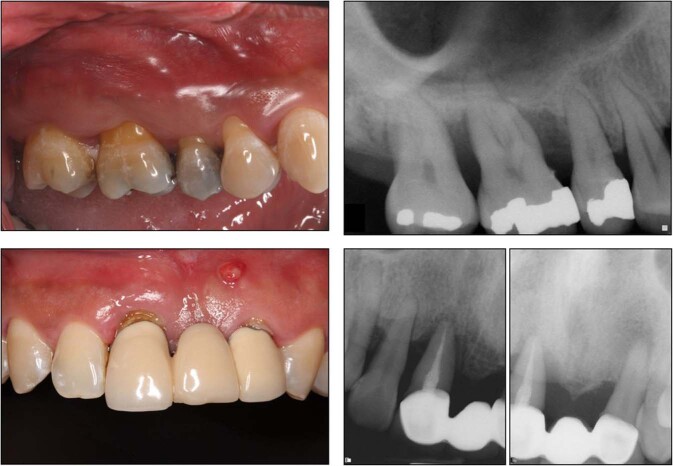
Clinical and periapical radiographic images of a patient with periodontal disease showing gingival inflammation and alveolar bone resorption
